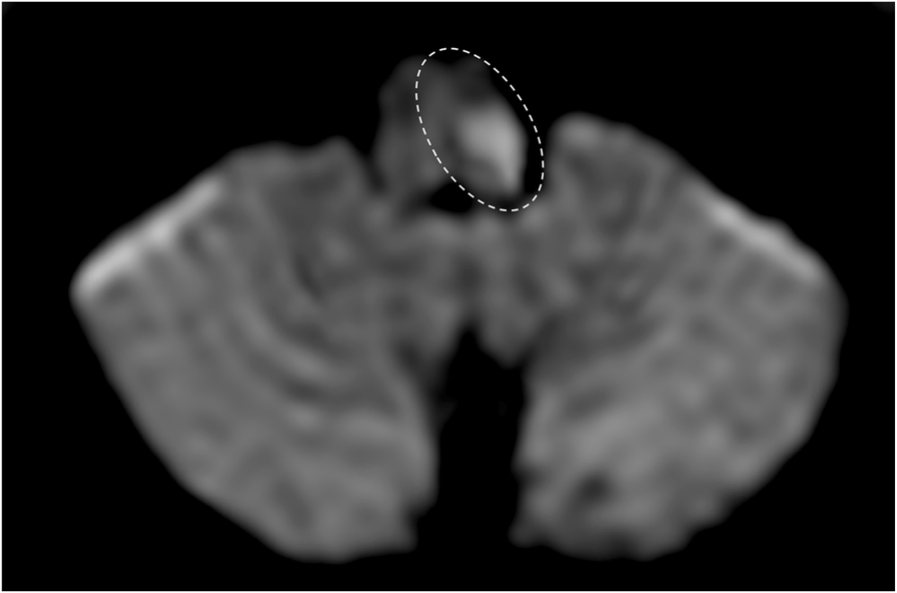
Wallenberg syndrome. Patient with left sensory loss, gait ataxia, nausea, and vertigo. MRI DWI (b1000) image demonstrates area of diffusion restricted in the left dorsal medulla oblongata, suggestive for recent ischemic lesion (dotted circle)
